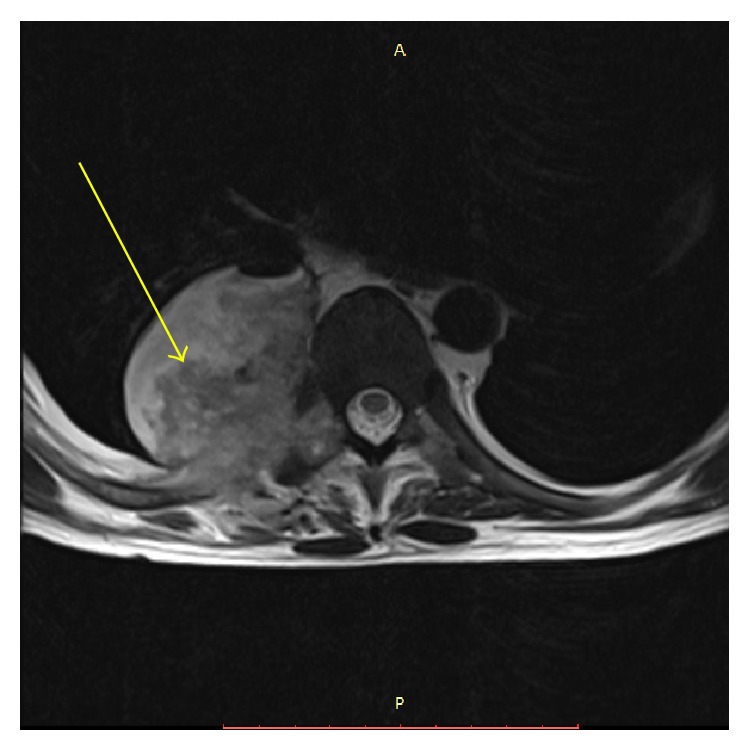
MRI spine (axial T1 and T2) showing paraspinal abscess on the right side with an air fluid level (arrow).
